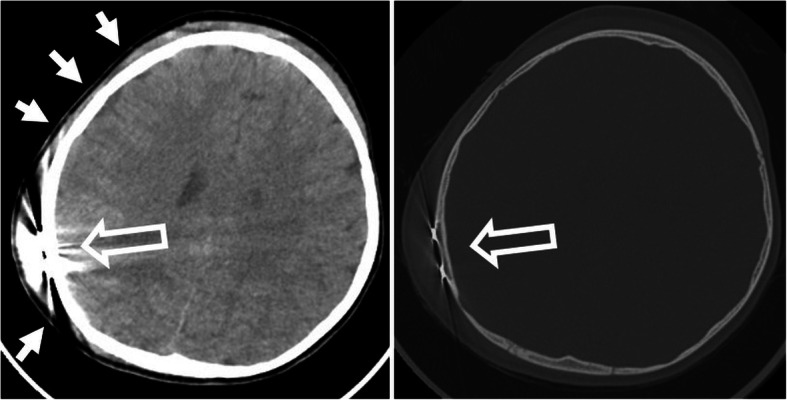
A 4-year-old male patient, with pain and insufficient hold of the speech processor after running into his brother. CT axial soft kernel image (left image) shows marked hematoma (arrows) in the skin at the frontal bone and temporal bone around the implanted magnet (hollow arrow). No intracranial bleeding. CT bone kernel axial image (right image) shows intact bone. The magnet is in the correct position
